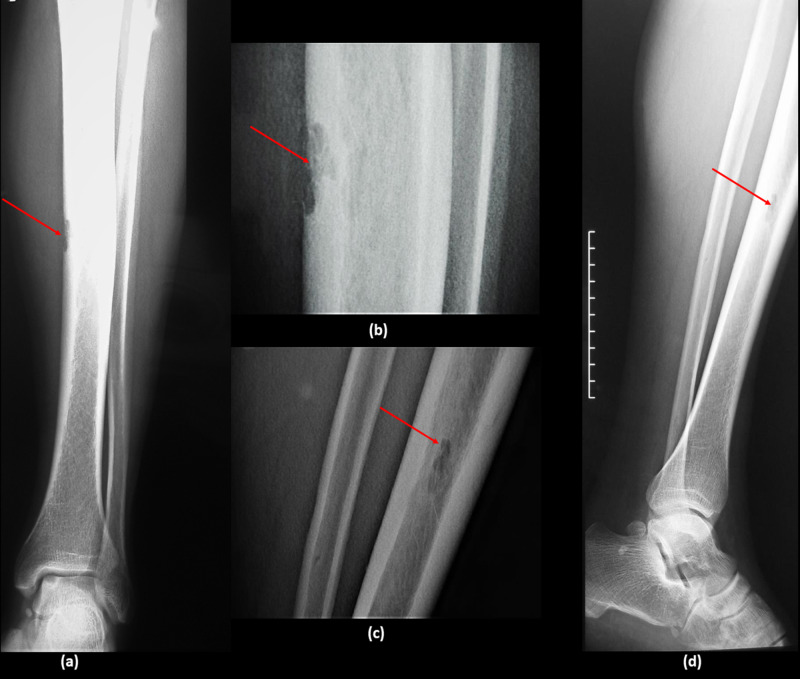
Radiograph of the leg Anteroposterior (a, b) and lateral (c, d) radiographs of the left leg showing evidence of a lytic, geographic, subperiosteal lesion on the medial anterior aspect of the mid-tibia (red arrows)
